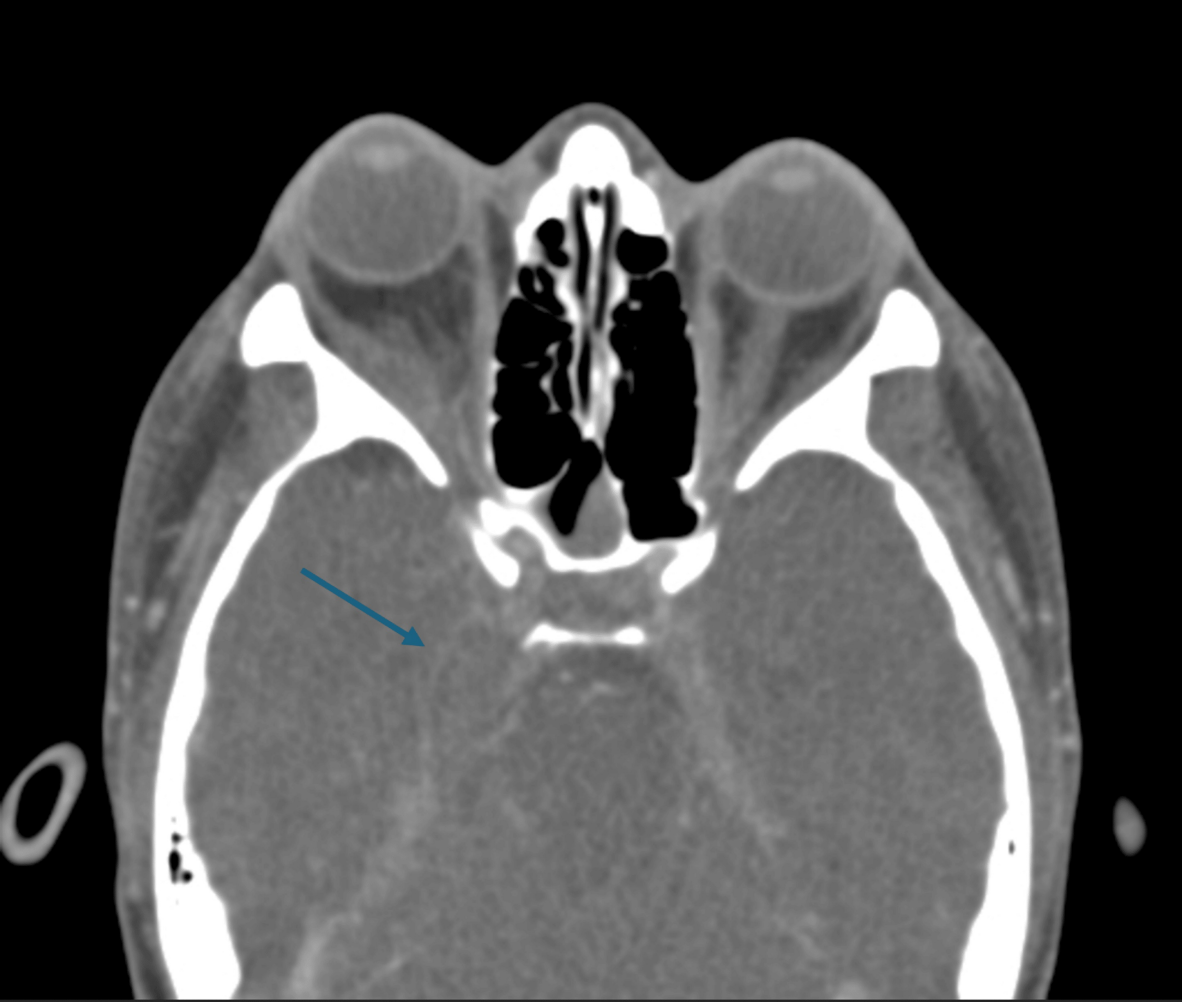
Axial CT scan of the superior orbit. The blue arrow points to the peripheral enhancing collection along the medial aspect of the right middle cranial fossa, measuring at least 3.2 cm x 1.4 cm.
